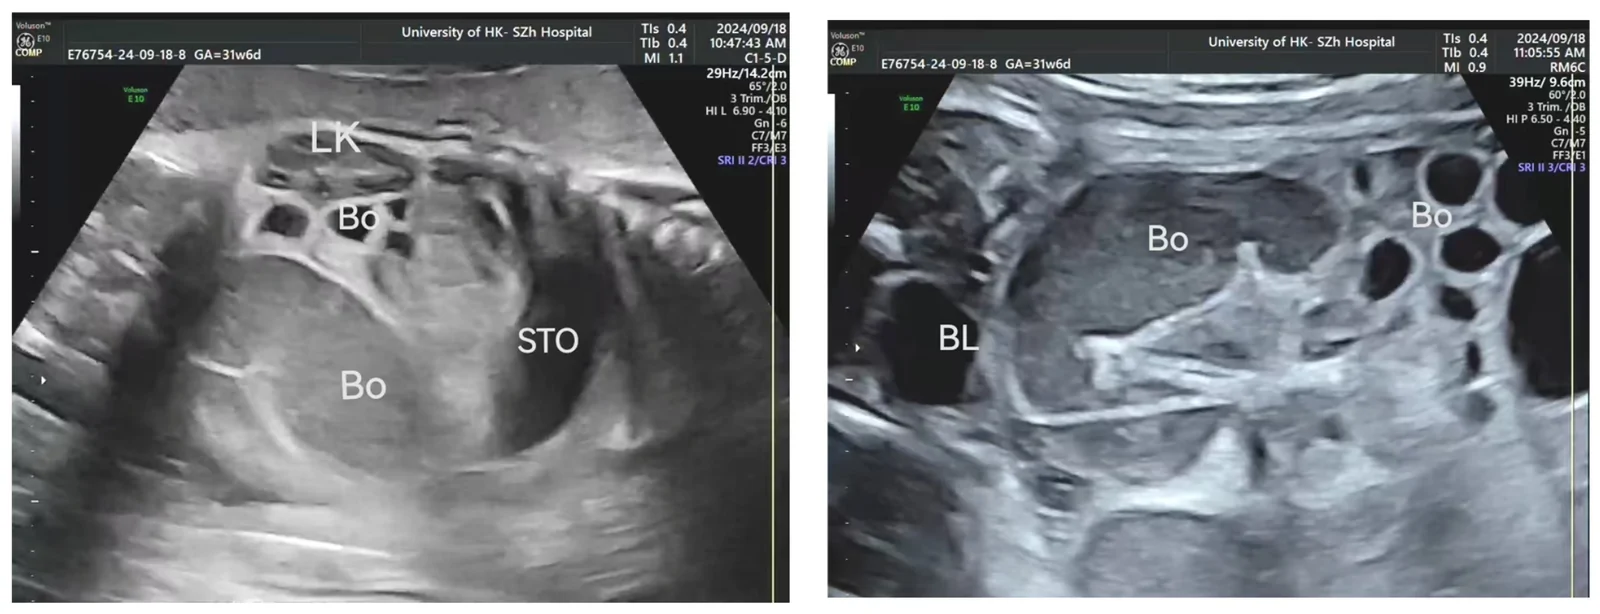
Fetal ultrasound (31 weeks): Longitudinal section (left image) and coronal section (right image) show dilated fetal bowel. STO: stomach; Bo: bowel; LK: left kidney; BL: bladder.
